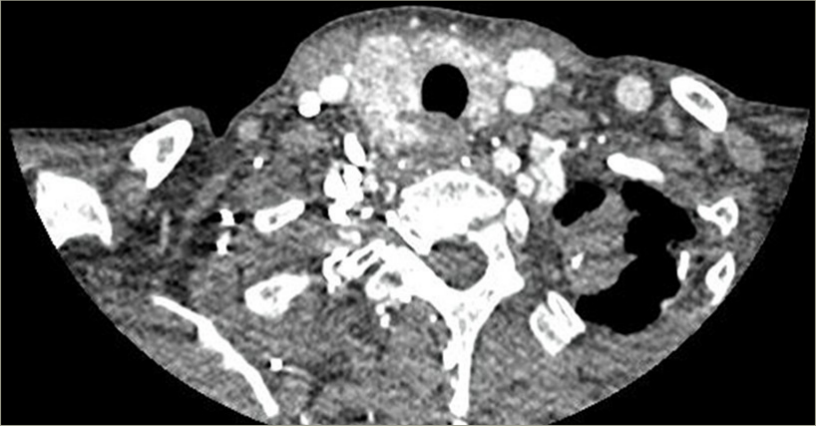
Computed tomography of the neck, case 3
